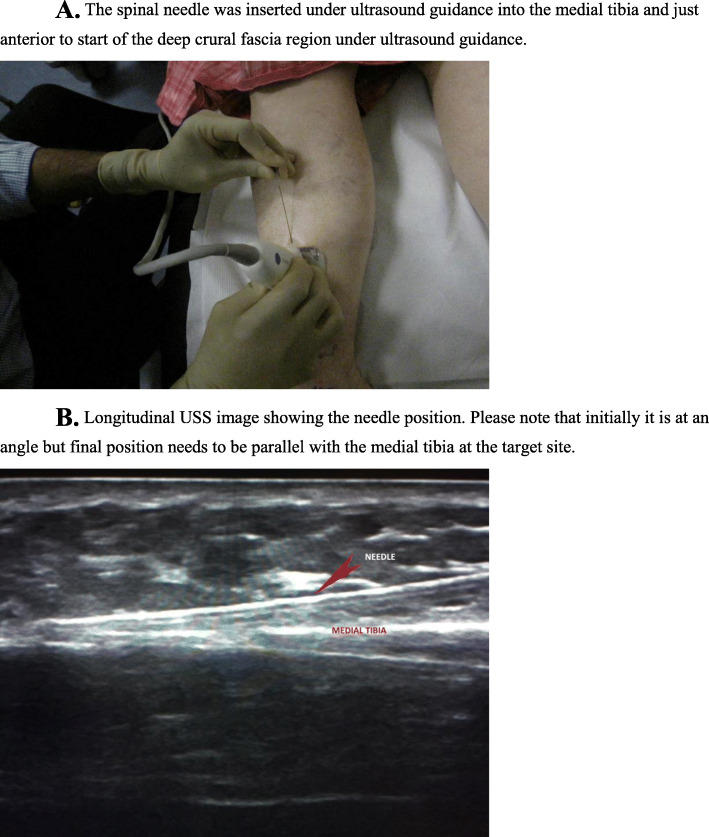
A. The spinal needle was inserted under ultrasound guidance into the medial tibia and just anterior to start of the deep crural fascia region under ultrasound guidance. B. Longitudinal USS image showing the needle position. Please note that initially it is at an angle but final position needs to be parallel with the medial tibia at the target site
